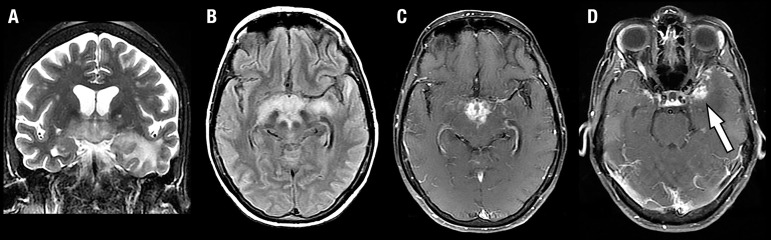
Case 1: Initial MRI. Coronal T2-weighted [A], axial FLAIR [B], and axial
